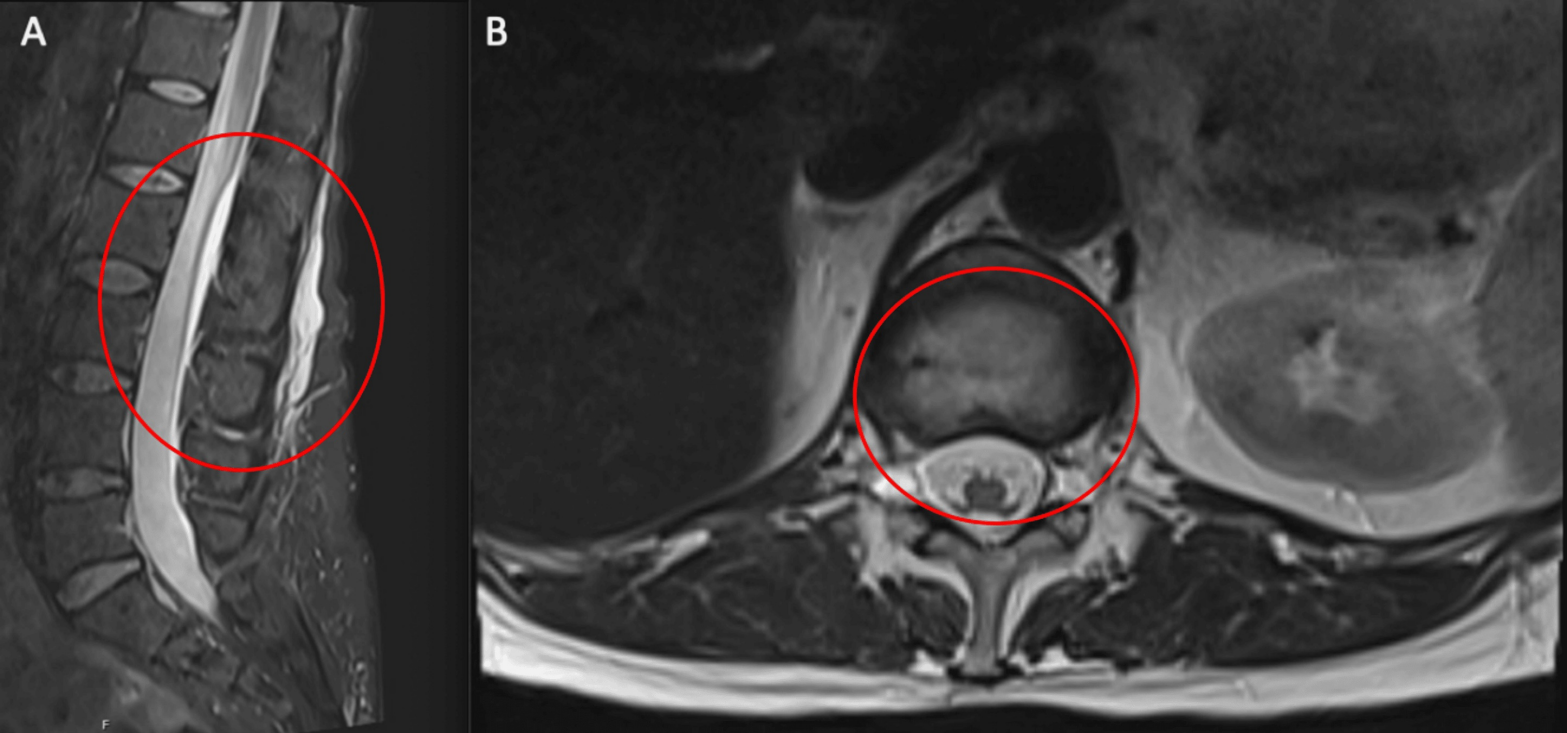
Sagittal and axial T2 MRI pan spine and follow-up imaging. A) Sagittal T2-weighted MRI pan spine demonstrating a large perineural cyst at the right T12-L1 level (red). B) Follow-up axial T2-weighted MRI two days later demonstrating the large perineural cyst at the right T12-L1 level (red).
